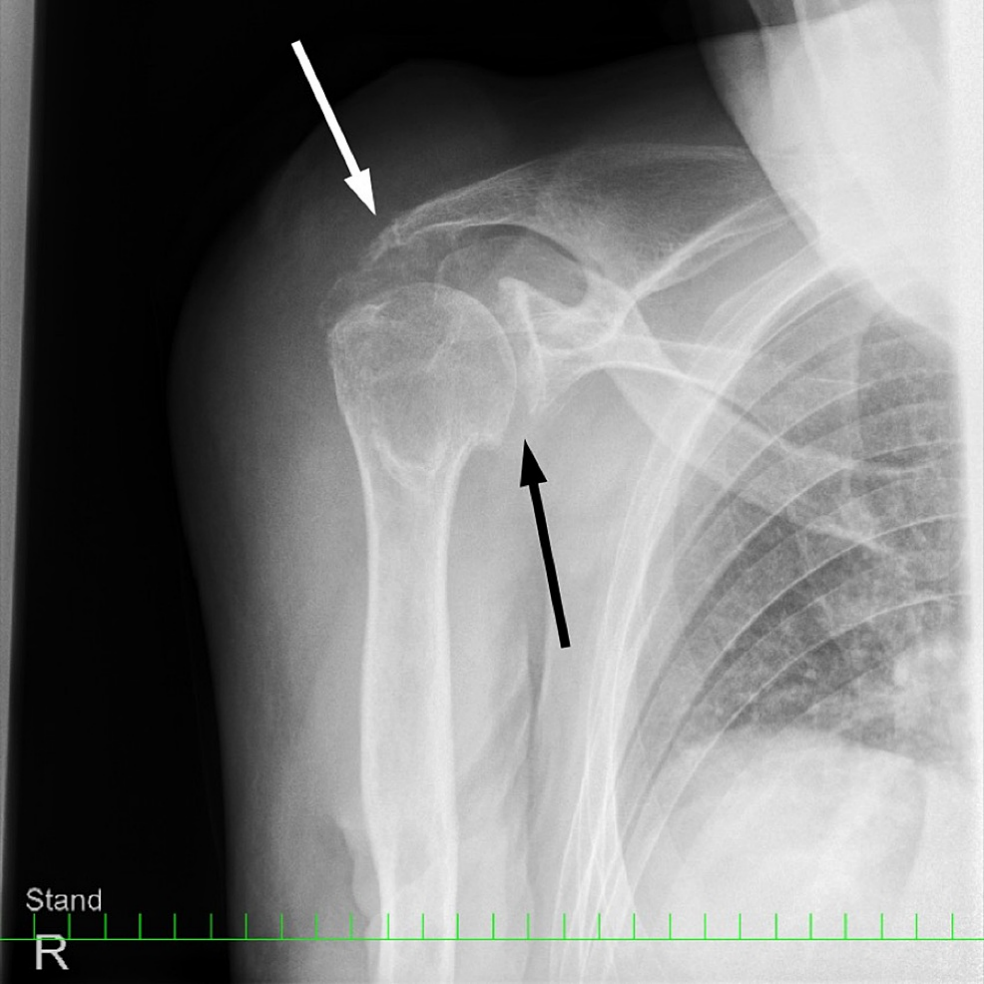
X-ray at presentation Degenerative changes in the glenohumeral joint (black arrow) and acromioclavicular joint (white arrow) with bone cysts of the humeral head.
